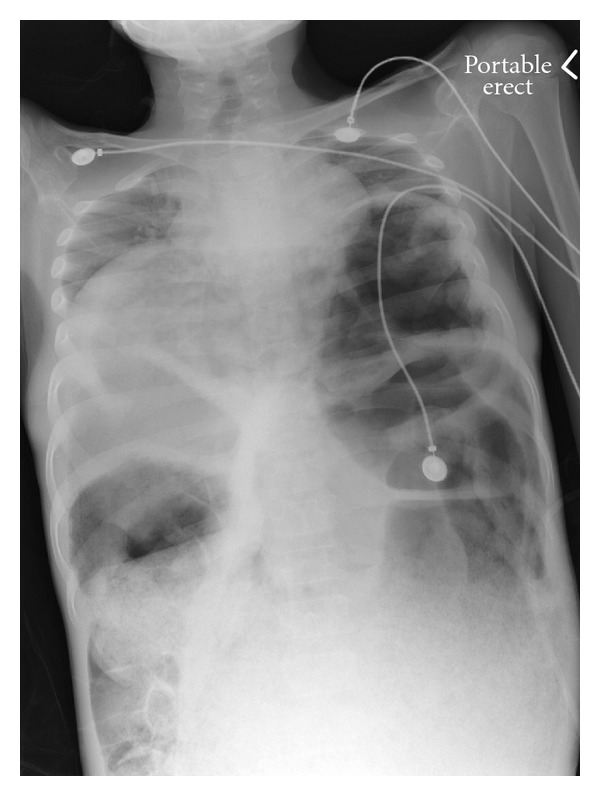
Chest X-ray performed just prior to intubation. Marked elevation of the heart and diaphragm with decreased lung volumes and compressed trachea.
